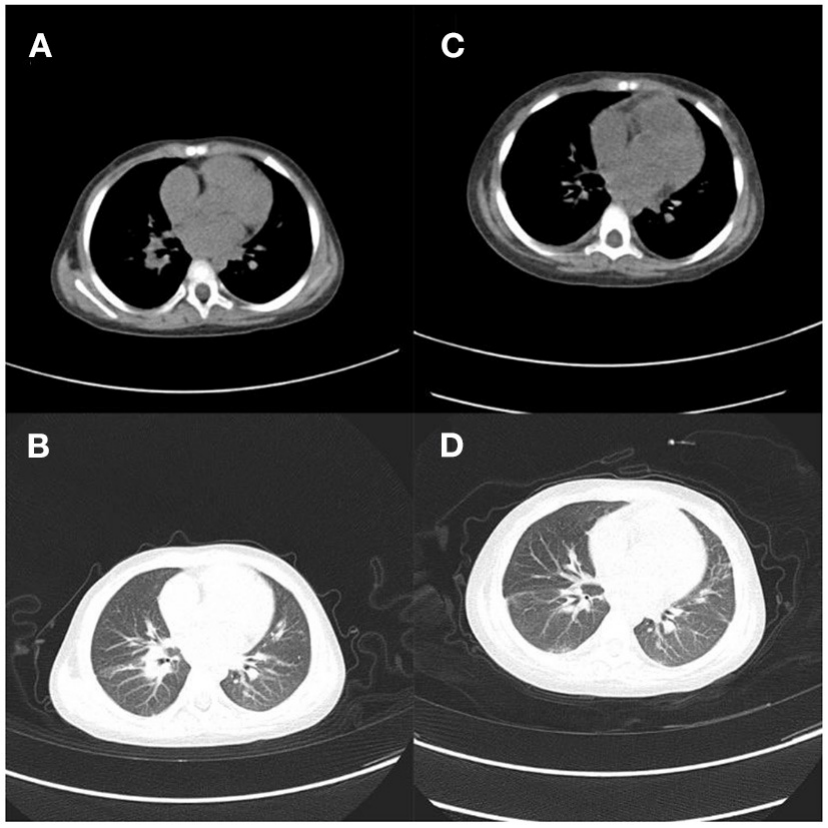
Case 3. (A,B) Lung CT on day 1 showed mild lung infection, multiple nodules and thickening of the anterior mediastinal soft tissue; (C,D) Chest CT showed worsened infection in both lungs, multiple small nodules, and new bilateral pleural effusion 2 months later.
